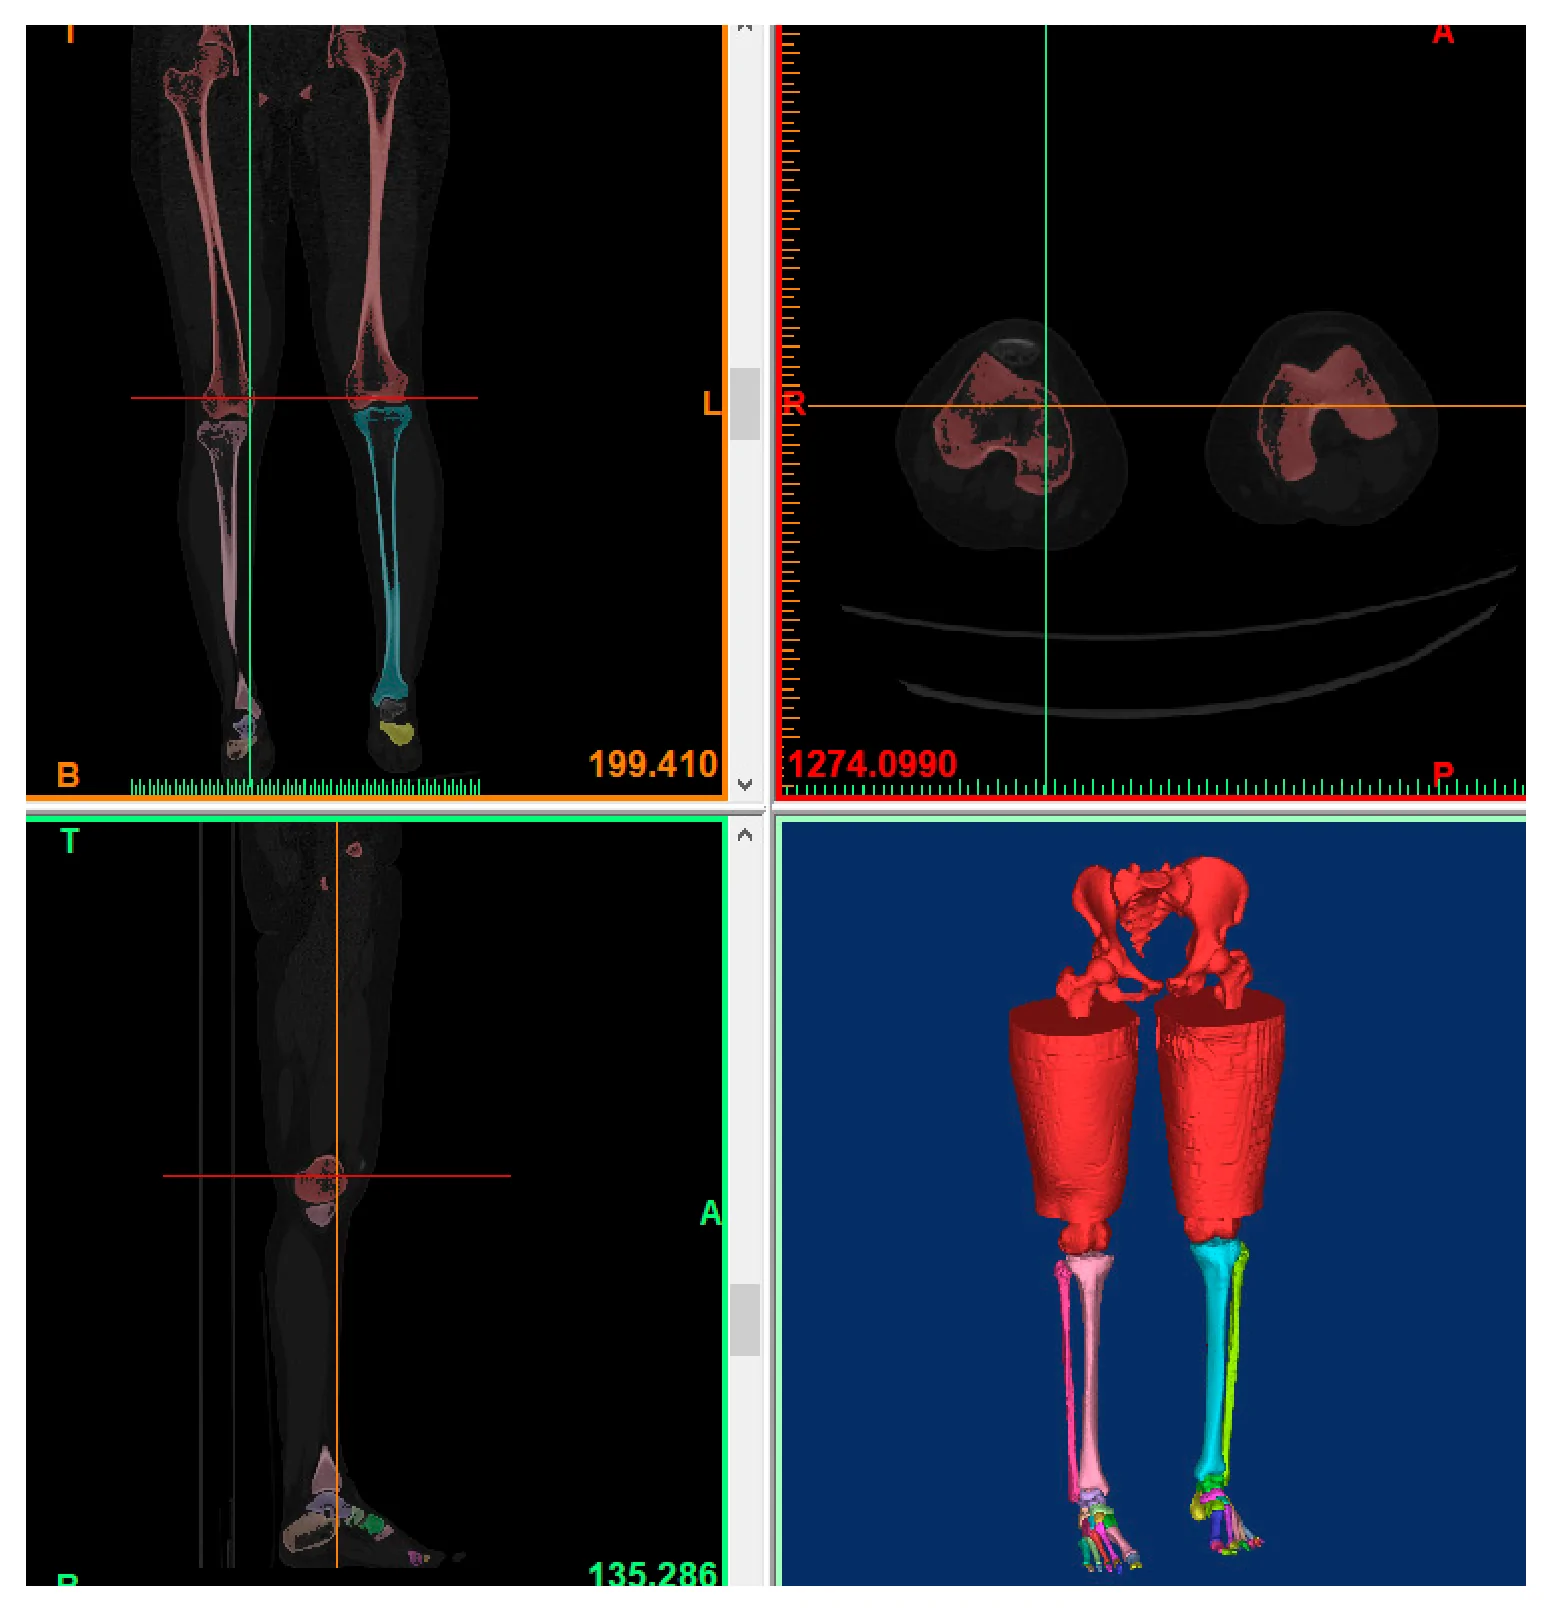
The 3D lower limb model containing bone and soft tissue based on CT images.
